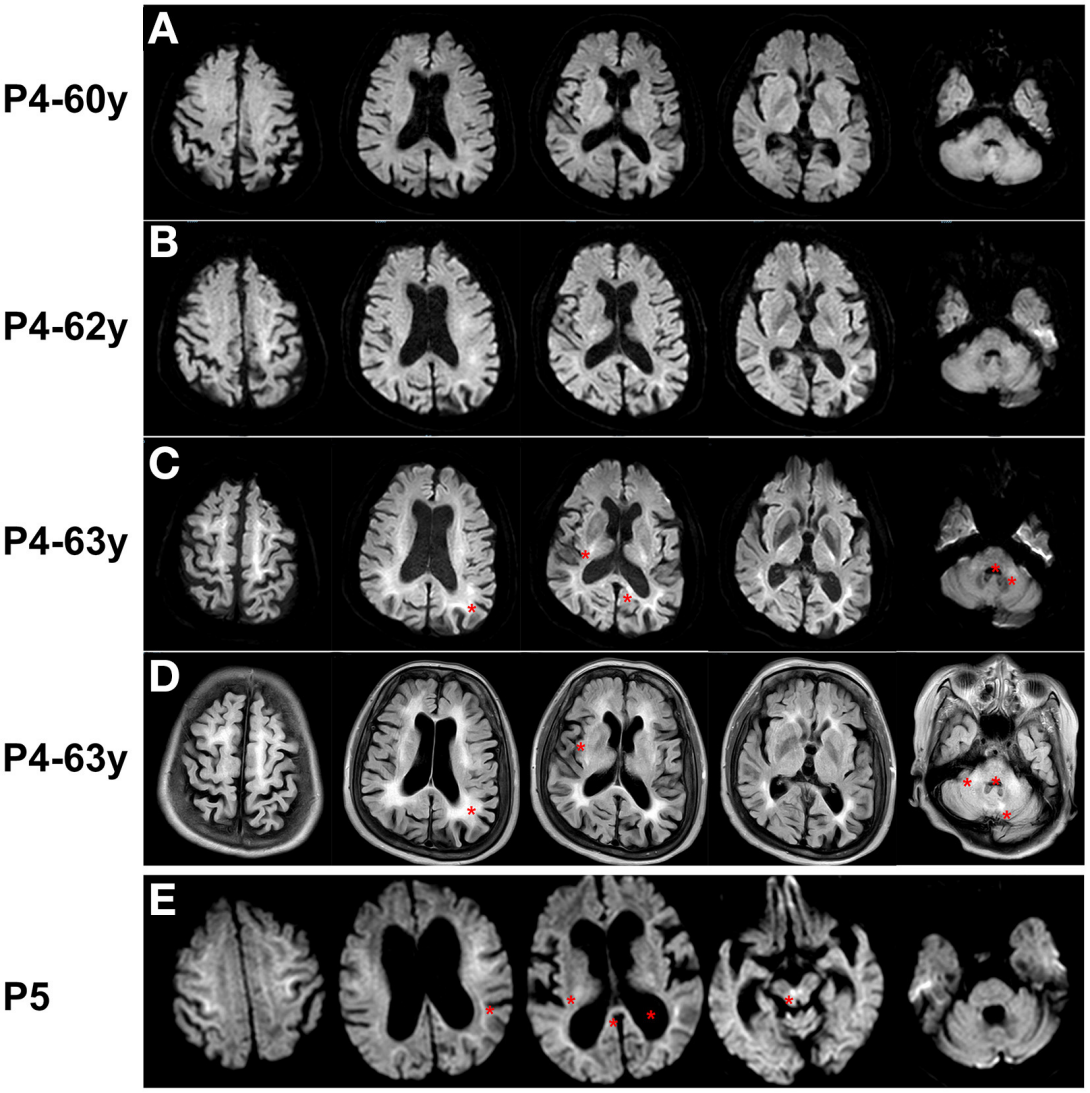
Brain MRI of Patient 4 and Patient 5. (A–C) DWI sequence of Patient 4 at age 60, 62, and 63 years showed irreversible hyperintensity in the corticomedullary junction in frontal, parietal, temporal, and occipital lobes, peri-globus pallidus, left splenium of the corpus callosum, deep lobar white matter of the corona radiata, pons, and branchium pontis. (D) FLAIR sequence of Patient 4 at age 63 years revealed hyperintensity corresponding with Fazekas grade 3 staging in subcortical regions, corpus callosum, external capsule, pons, branchium pontis, and paravermal areas of cerebellum. (E) DWI sequence of Patient 5 showed ribbon sign in frontal, parietal, temporal, and occipital lobes, and high-intensity signals in thalamus, splenium of the corpus callosum, and the, and dilated ventricles (asterisks).
